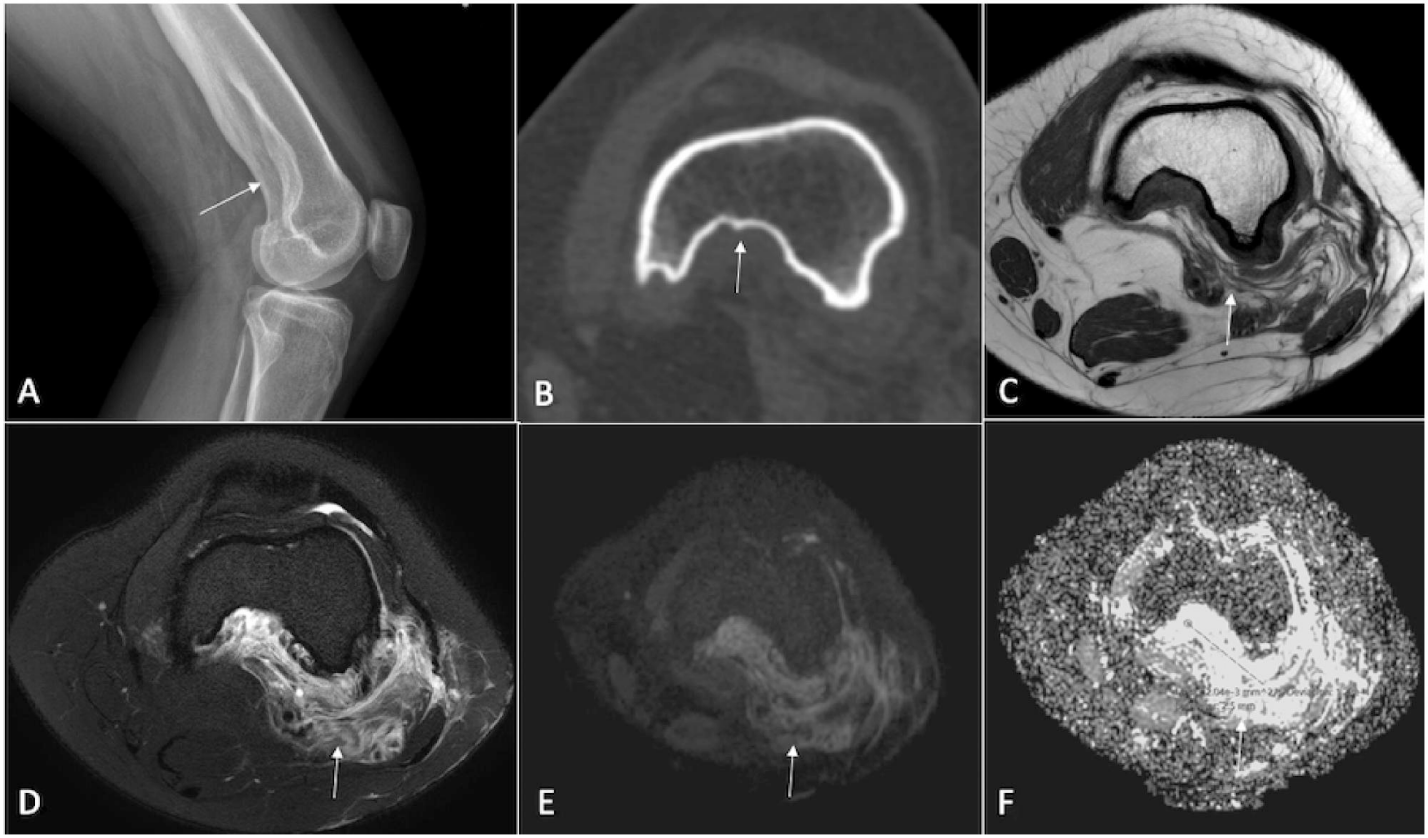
An 18-year-old female with a history of neurofibromatosis type 1 (NF1) presents with deep, stabbing pain in her left lower extremity, radiating from the knee to the foot, along with weakness. Radiograph (A) reveals scalloping (arrow) of the posterior femoral cortex. A CT axial section shows smooth scalloping in the posterior femoral cortex (arrow). The axial T1-weighted image displays a heterogeneously hypointense mass (arrow) with areas of fat. The axial T2-weighted fat-saturated sequence shows a heterogeneously hyperintense mass (arrow) with fat suppression. DWI (E) indicates that the mass (arrow) is hyperintense with facilitated diffusion, displaying high ADC values (arrow) (F). The lesion was diagnosed as neurofibroma.
